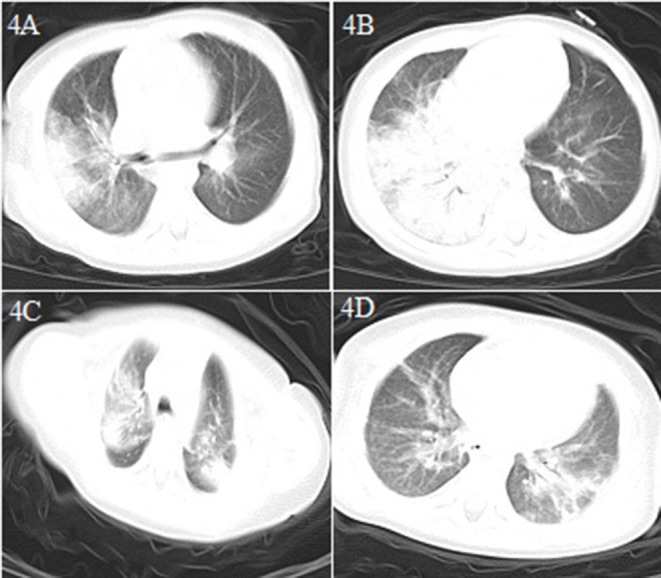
Chest CT images for case 4 showing a large dense shadow with some consolidation in the right lung and a few high-density shadows in the upper lobe of the left lung on day 6 after symptom onset (A,B) and patchy dense shadows mixed with ground-glass opacity in the bilateral upper lobe tip, right middle lobe, and left lower lobe, and air bronchogram inside on day 15 after symptom onset (C,D).
